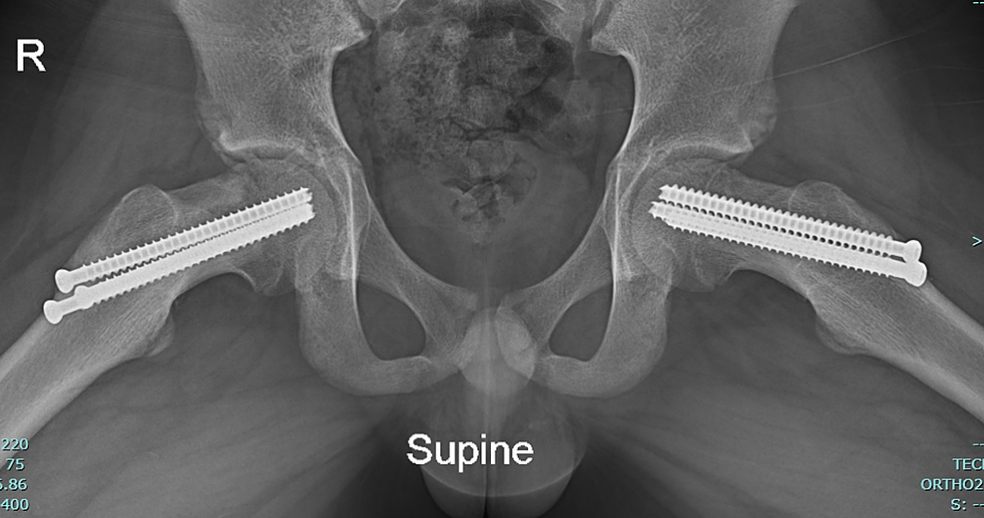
Bilateral frog leg radiographs at one year postoperatively.
